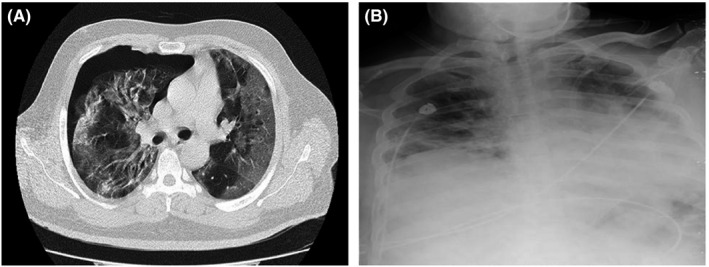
Chest CT scan and chest X‐ray of case 6
